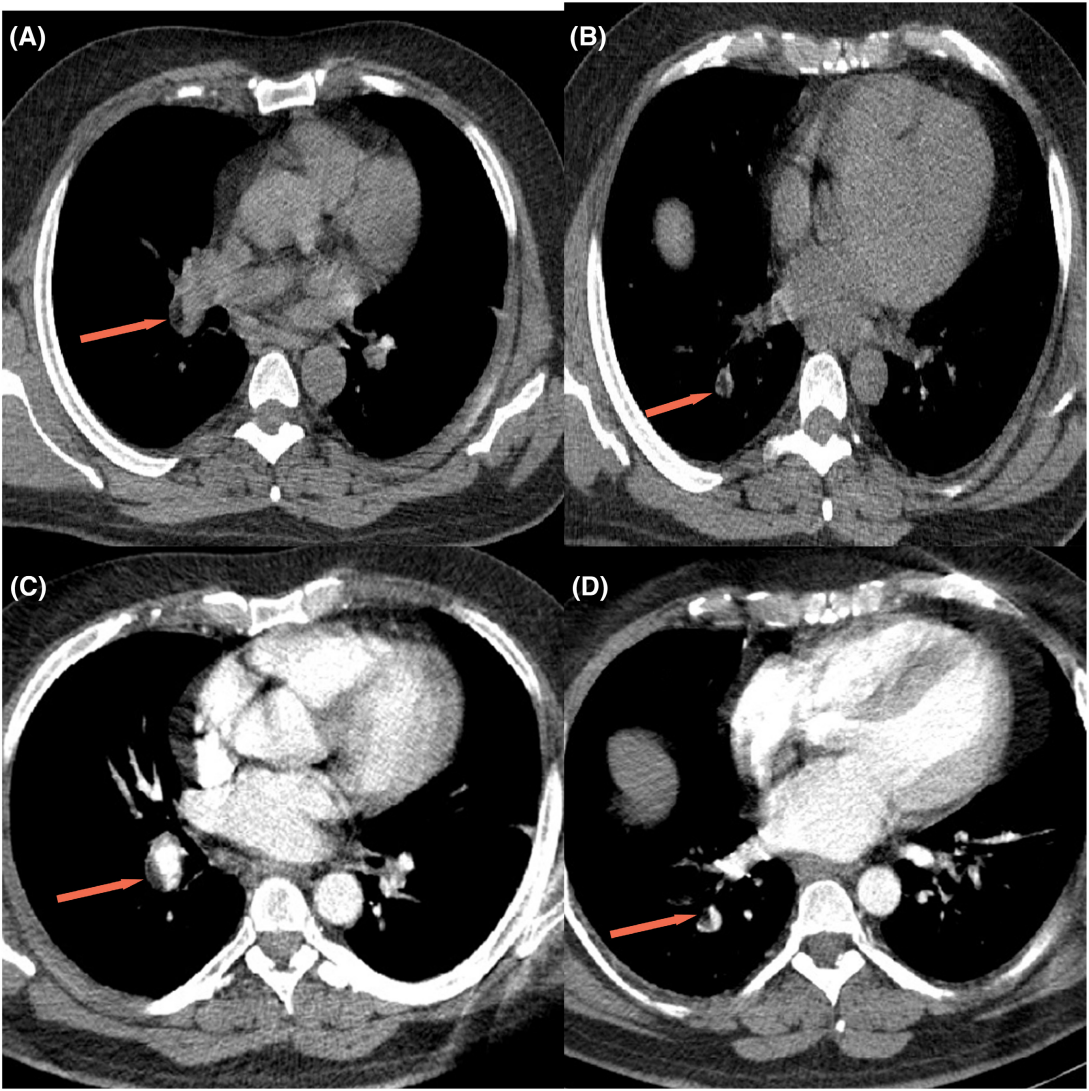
Non‐contrast chest CT scan (A, B) and pulmonary CT angiography (C, D) show intraluminal lesion with a fat density (arrows) in the right interlobar pulmonary artery, extending to the segmental branches of the right lower lobar, suggesting a fat embolism.
